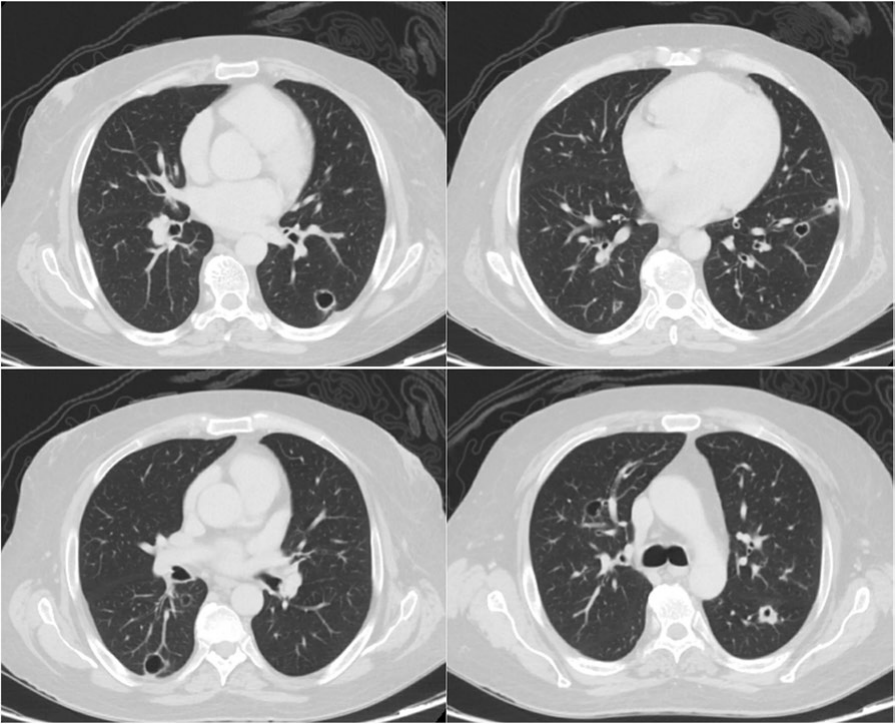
Axial sections of a contrast-enhanced CT scan of the chest show multiple bilateral cystic and cavitary lung lesions, predominantly involving the lower lobes. A cavitary nodule is seen in the superior segment of the left lower lobe showing thickened wall (*upper left image*)
